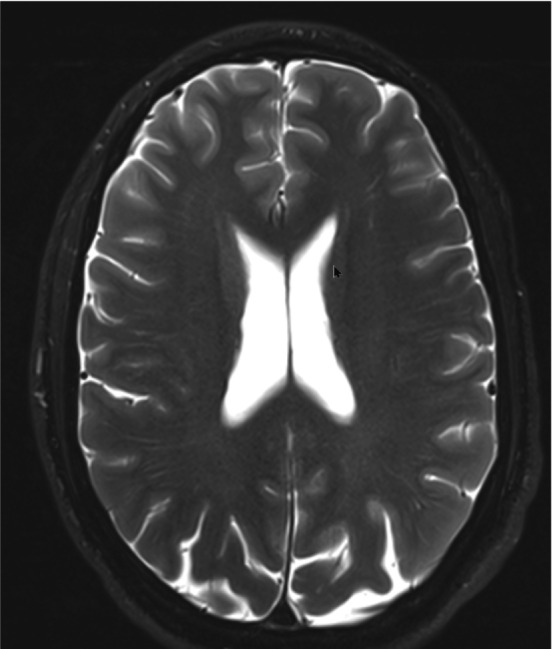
Example of enlarged VR spaces. T2 FLAIR image showing enlarged VR spaces mainly in the posterior quadrants.
